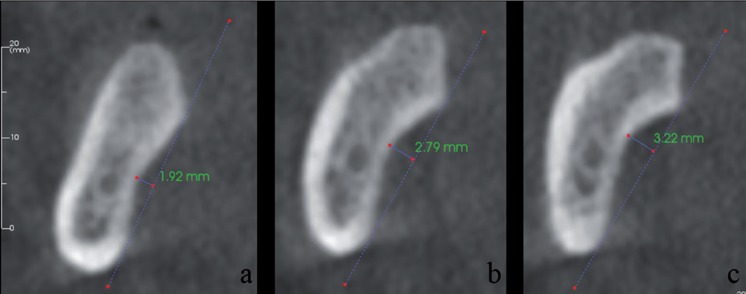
Types of submandibular fossa on cross-sectional CBCT images. Type I, concavity < 2 mm; Type II, concavity = 2 – 3 mm; and Type III, concavity >3 mm.
